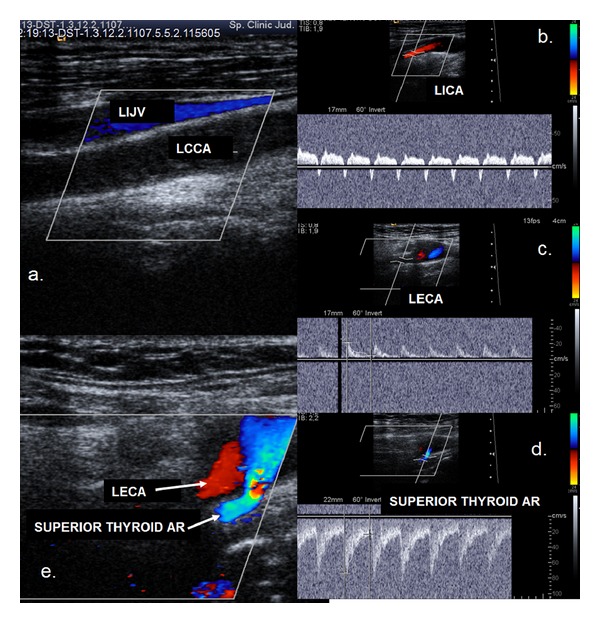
Ultrasound examination showing common carotid artery occlusion with patent distal vessels. Anterograde flow in both the ICA and ECA. (a) Colour mode examination: no flow in the left common carotid artery (LCCA), and the vessel lumen is filled with thrombotic material. (b) Duplex mode examination: anterograde flow in the left internal carotid artery with a steal effect, deceleration, and inversed flow in mesosystole. (c) Duplex mode examination: anterograde flow in the left external carotid artery. (d) Duplex mode examination: retrograde flow in the left superior thyroid artery. (e) Colour mode examination: retrograde flow in the left superior thyroid artery and anterograde flow in LECA.
